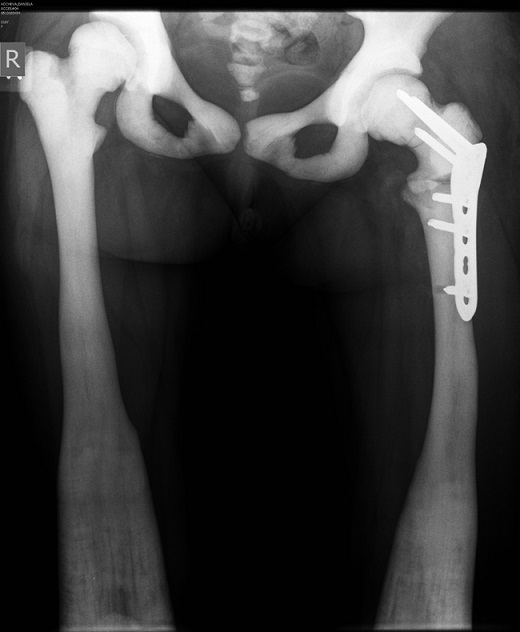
Postoperative roentgenographs – ORIF with 120° LCP Pediatric hip plate
